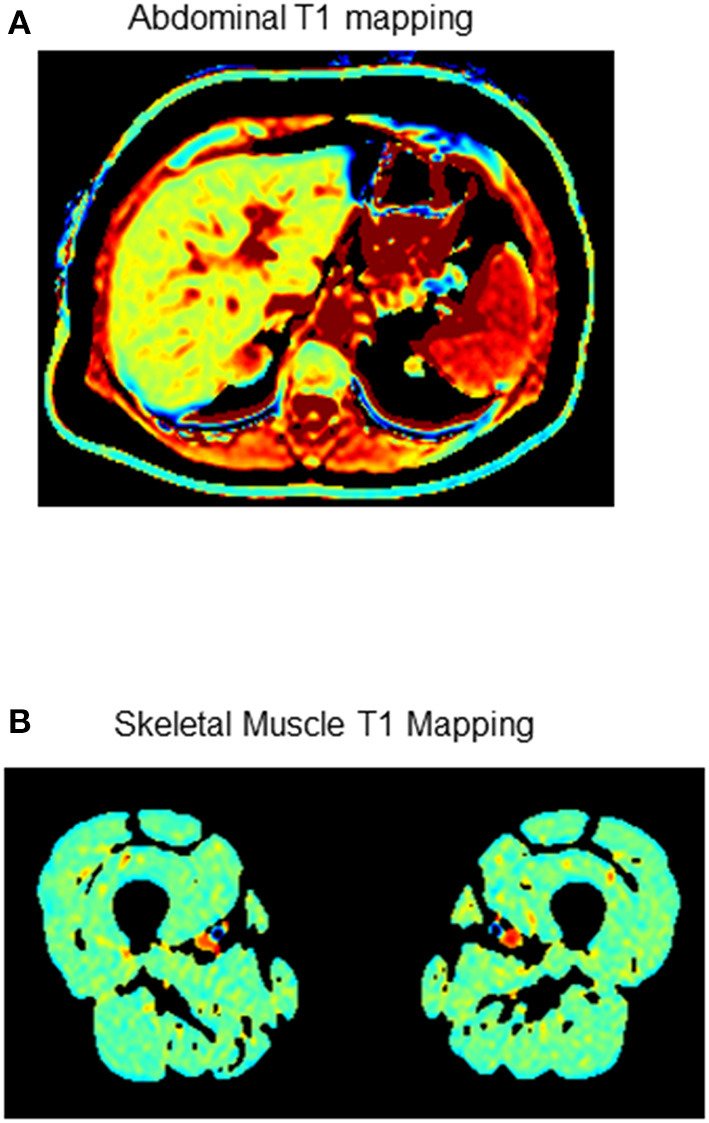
Examples of MRI T1 mapping sequences to assess the extra-cardiac effects of exercise training in patients with or at risk for cardiovascular disease. (A) Abdominal fat density (B) Skeletal muscle fat compartments. Dark signal = fat.
